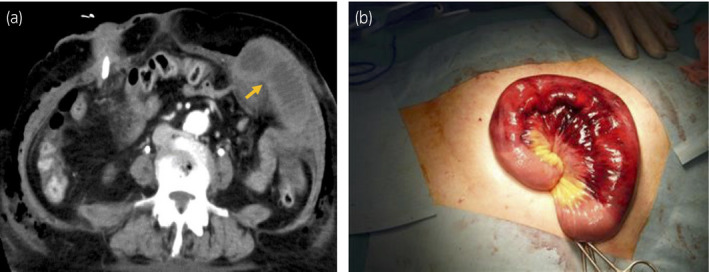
(a) Computed tomography showed the small intestinal herniation from the left port site. The arrow shows hernial orifice. (b) Surgeons showed engorgement of the small intestine and discoloration of bowel loops.
